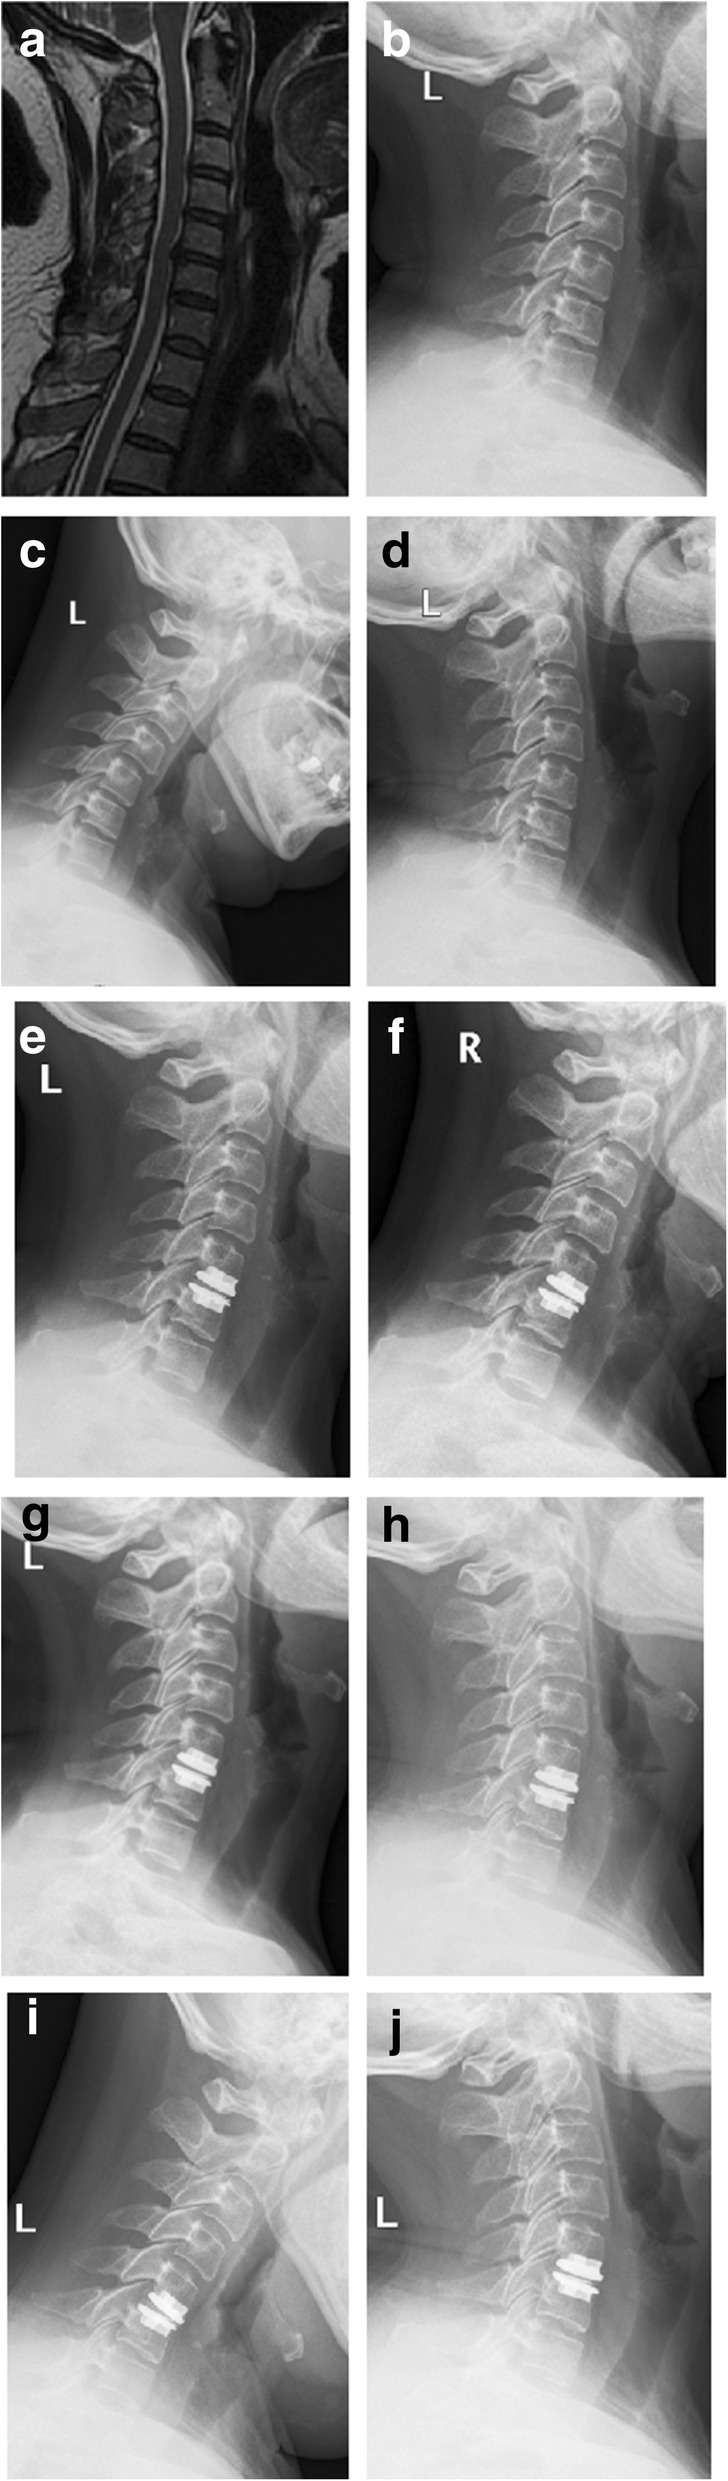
The primary outcomes before and after C5–6 TDR on a 54-year-old female. C4–5 is UAS and C6–7 is LAS. a C5-6 CDDD on the T2-weighted image of MRI before TDR; b–d Neutral lateral X-ray and flexion-extension X-ray before TDR. ROM, LOR, and IDH of C5–6 are 8.6°, − 2.3° and 3.76 mm, respectively; The primary outcomes of C4–5 and C6–7 are 9.7°, 8.5°, 4.41 mm and 5.7°, − 3.6°, 3.37 mm, respectively. e–g Neutral lateral X-ray and flexion-extension X-ray 1 week after TDR. ROM, LOR, and IDH of C5–6 are 7.3°, − 3.5°, and 6.85 mm, respectively; the primary outcomes of C4–5 and C6–7 are 6.3°, 4.8°, 4.19 mm and 4.8°, − 2.6°, 3.85 mm, respectively. h–j Neutral lateral X-ray and flexion-extension X-ray 10 years after TDR. ROM, LOR, and IDH of C5–6 are 9.0°, − 2.7°, and 6.55 mm, respectively; The primary outcomes of C4–5 and C6–7 are 8.1°, 7.0°, 4.61 mm and 6.5°, − 4.1°, 3.84 mm, respectively. There is no statistical change on primary outcomes of C4–5 and C6–7 although a higher IDH of C5–6 after TDR.
